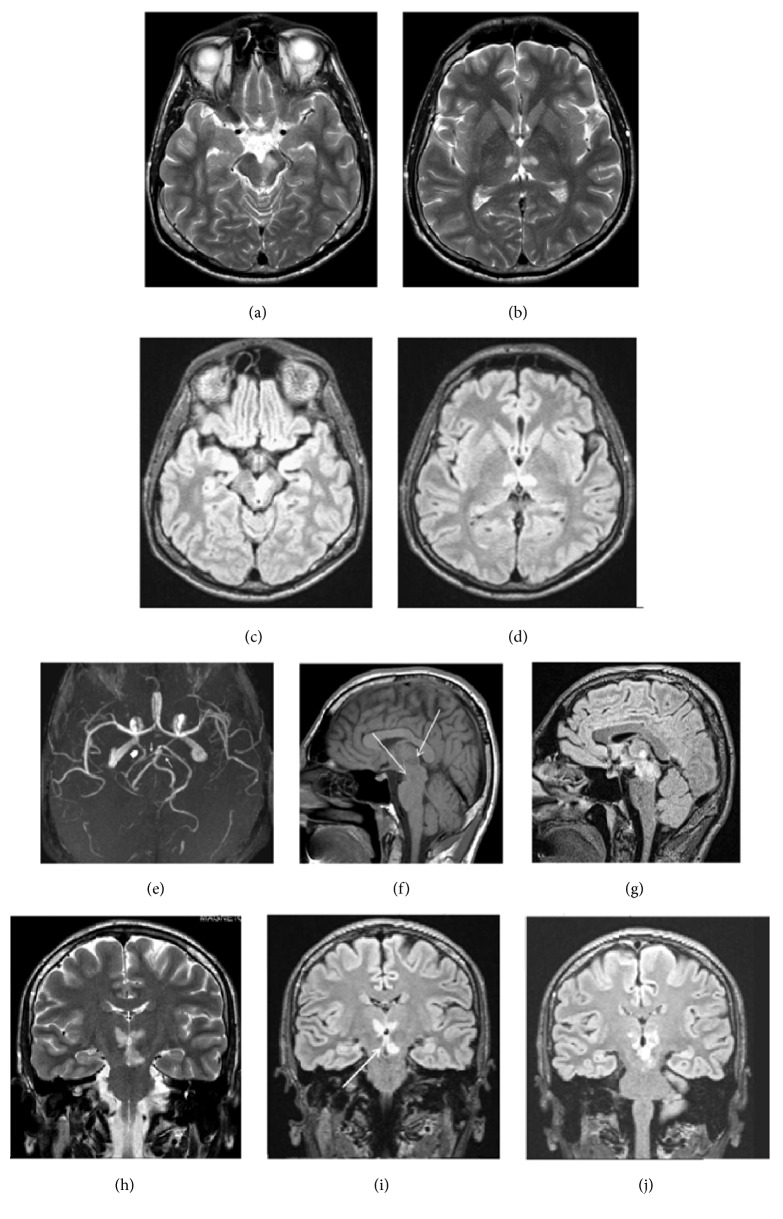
(a) Axial sections of T2-weighted images obtained 20 hours after the onset of symptoms showed areas of increased signal intensity in the left mesencephalon and (b) paramedian thalamic nuclei. (c, d) Axial brain FLAIR images. Hyperintense signals in the rostral midbrain and the paramedian thalamic suggest acute Percheron artery infarction. (c) “V-shaped” hyperintense signal along the pial surface of interpeduncular fossa in midbrain. The mesencephalon lesion extends to the periaqueductal gray matter. (e) MR angiography shows a right-sided FPCA (thick white arrow), arising directly from the ipsilateral internal carotid artery. Patency of the basilar artery and tip, left PCA, and posterior communicating artery; the thin arrows indicate the superior cerebellar arteries, with normal appearance. (f) Sagittal section, T1-weighted: showed ill-defined areas of hypodensity in the thalamopeduncular junction (white arrows). (g) Sagittal section, FLAIR: hyperintense images with the same topography. Coronal sections on T2 (h) and FLAIR images (i, j). Relatively symmetric hyperintense signals in the paramedian inferior thalami, extending (asymmetrically) into the medial and rostral mesencephalon (territory of the artery of Percheron). (i) Coronal section, FLAIR: “lambda-shaped” (Λ) hyperintense signal, adjacent to the pial layer of the interpeduncular fossa, next to the infarction zones in the thalamic–mesencephalon junction, equivalent version of the “V-shape” observed in axial sections (c). (FLAIR, fluid-attenuation inversion recovery images; FPCA, fetal posterior cerebral artery.)
